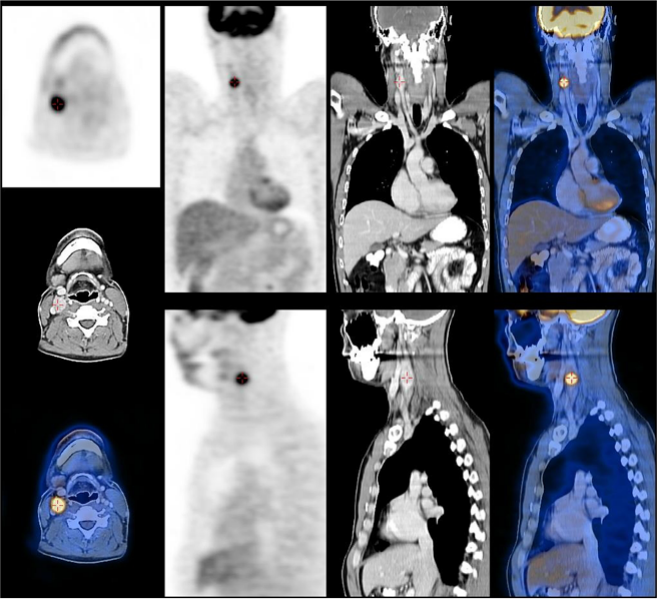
A positron emission tomography-computed tomography (PET-CT) scan showing the tumour of the neck region III with increased uptake.
